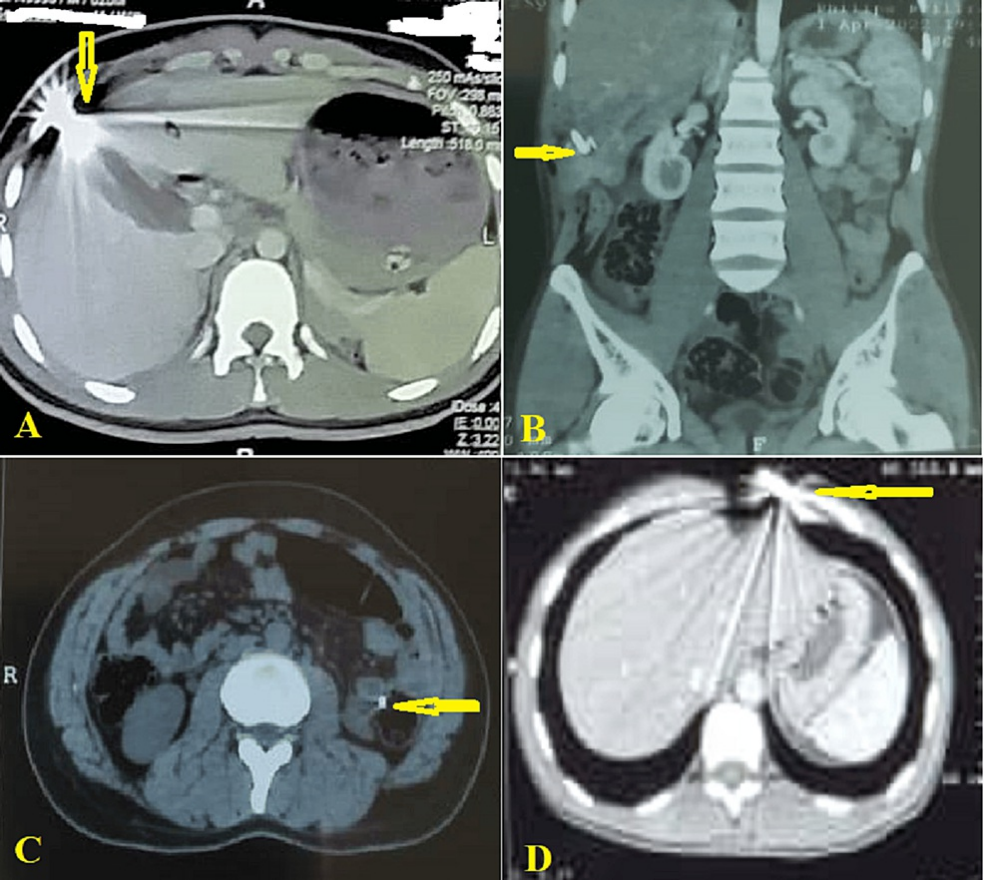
Computed tomography scan showing (A) penetrating gunshot abdominal injury with hemoperitoneum and liver damage (yellow arrow) (axial view), (B) penetrating gunshot abdominal injury with hemoperitoneum and liver damage (yellow arrow) (coronal view), (C) penetrating gunshot abdominal injury with a foreign body in the wall colon (yellow arrow) (axial view), and (D) penetrating gunshot thoracoabdominal injury (yellow arrow) and pneumoperitoneum (axial view)
